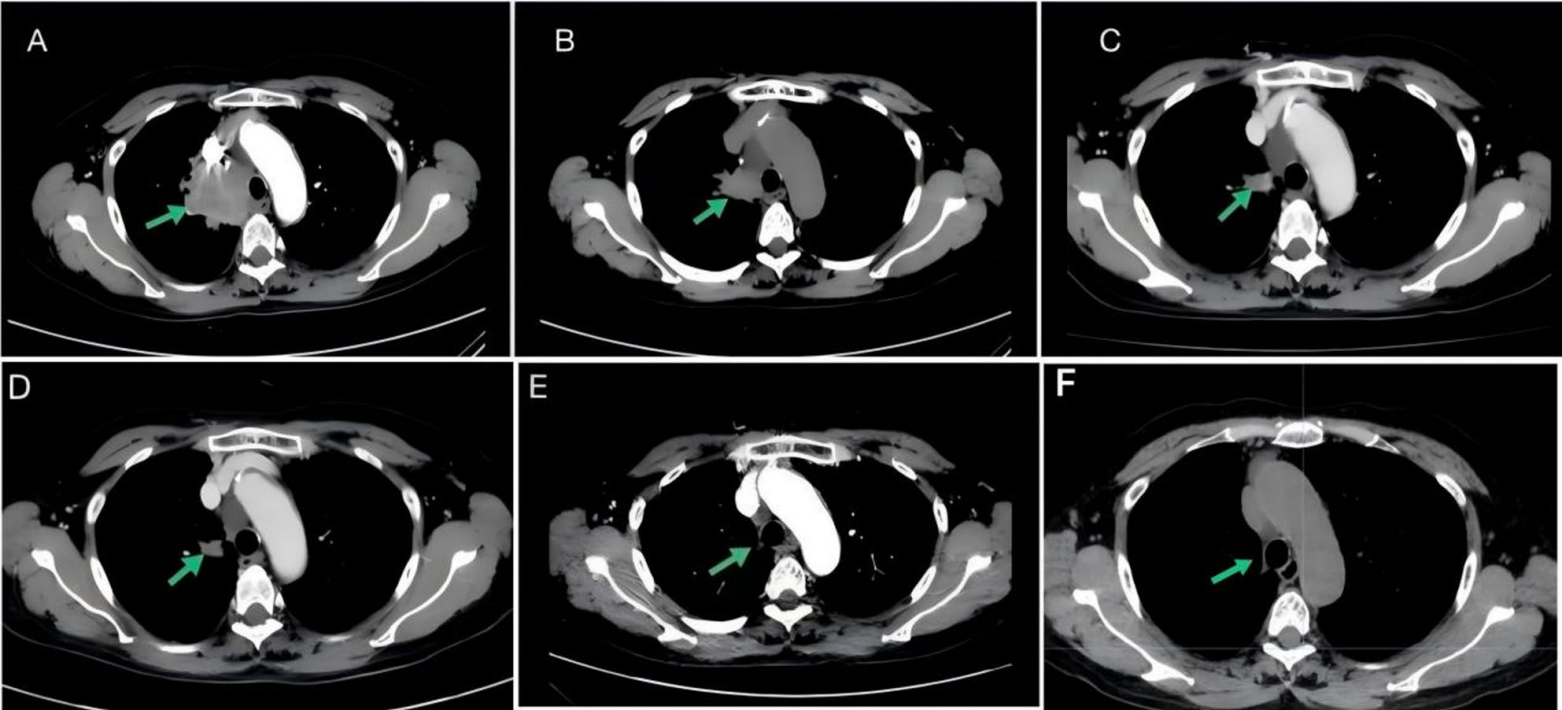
(A) Contrast-enhanced chest CT (May 9, 2024): A 55.5 × 43.5 mm soft-tissue mass adjacent to the right hilum with mediastinal lymphadenopathy (no distant metastasis). (B) Two weeks post-ensartinib (May 31, 2024): Primary lesion reduced to 32 × 24 mm; mediastinal lymph nodes regressed. (C) Week 8 (July 17, 2024): Lesion further reduced to 20 × 12 mm. (D) Week 12 (August 20, 2024): Residual lesion measured 12 × 11 mm (78.4% reduction from baseline). (E) Postoperative CT (April 10, 2025): No recurrence. (F) Postoperative CT (November 10, 2025): No recurrence
